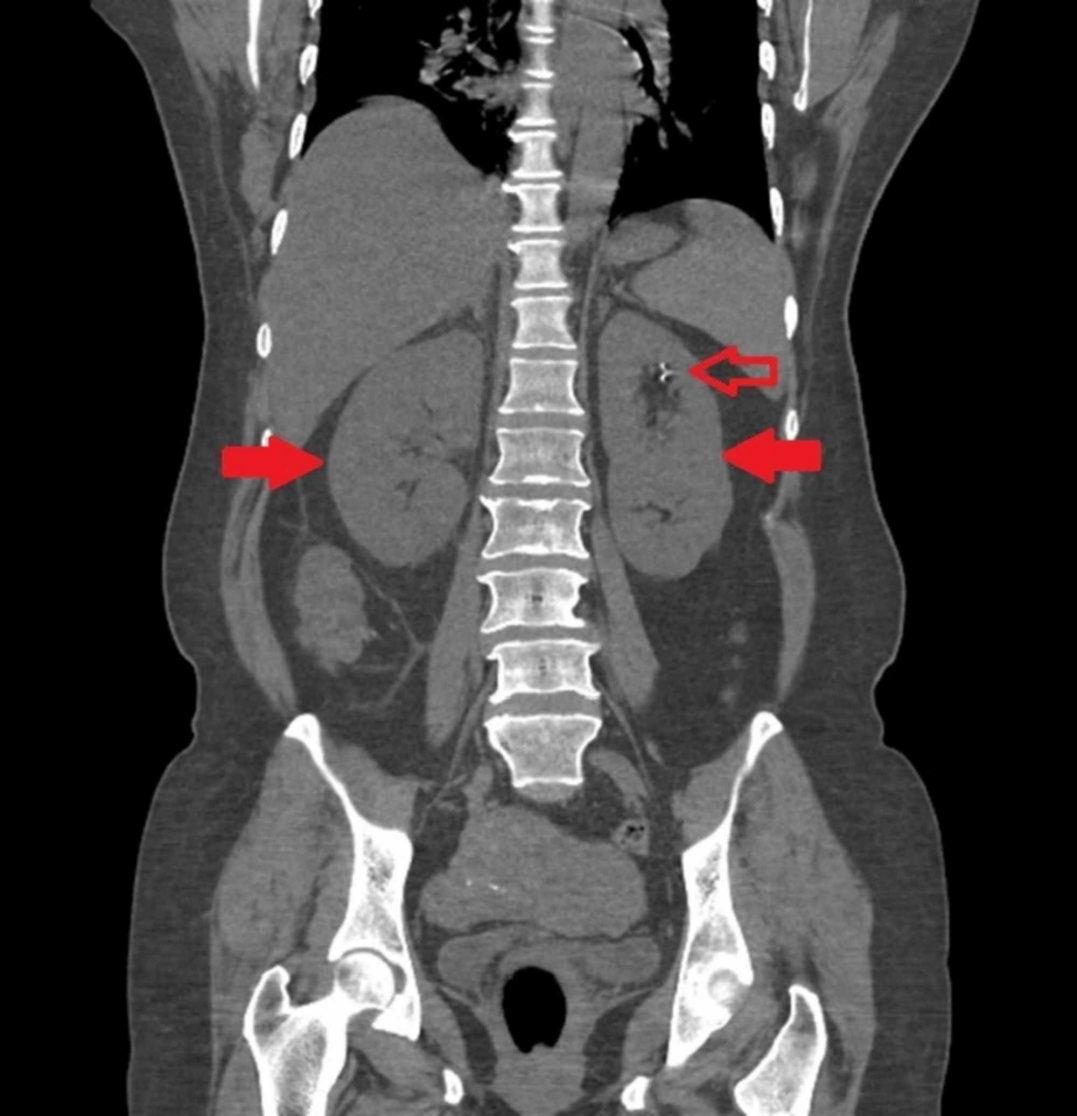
Computed tomography of the abdomen with contrast showing bilateral hydronephrosis (solid red arrows) with left non-obstructing renal calculi (red arrow)
